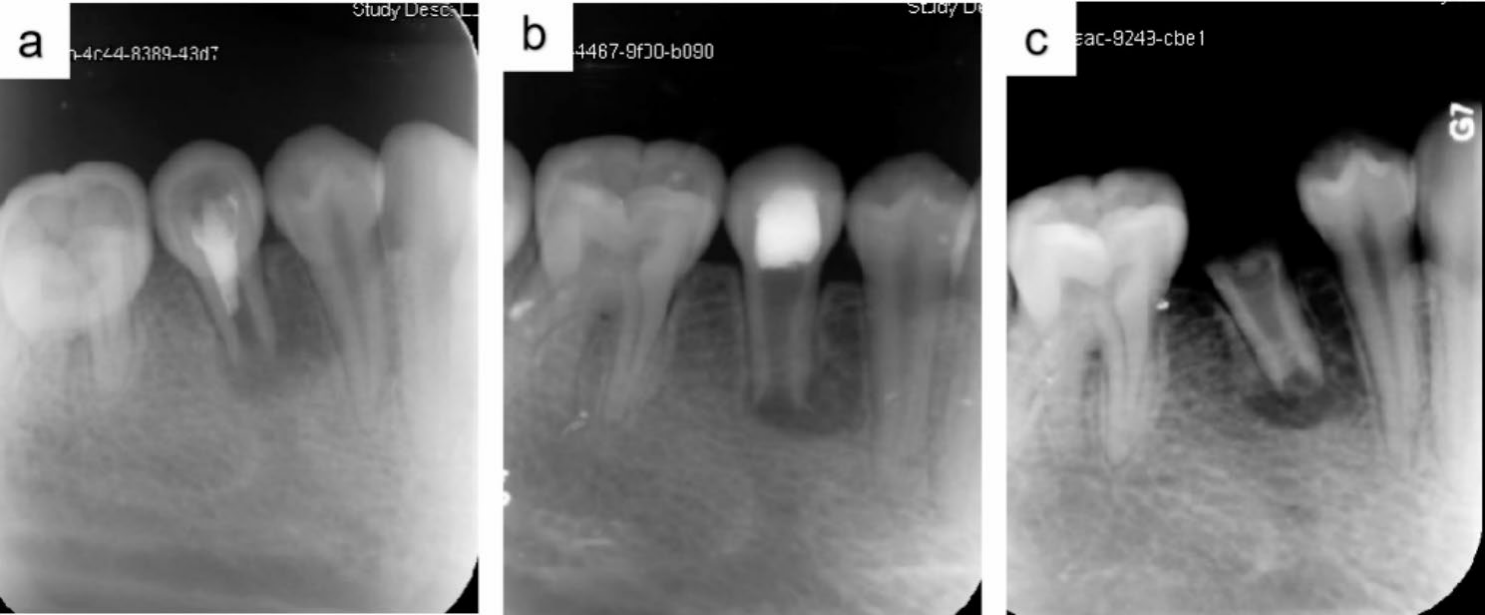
Periapical radiographs over the 16-month follow-up period. (a) Periapical radiograph of tooth 45 showing an incompletely developed root with a periapical lesion. (b) Postoperative periapical radiograph at the 9-month follow-up period showing no obvious resolution of the periapical lesion or healing of the periapical bone. (c) Postoperative periapical radiograph at the 16-month follow-up period showing sustained periapical lesion and crown fracture
